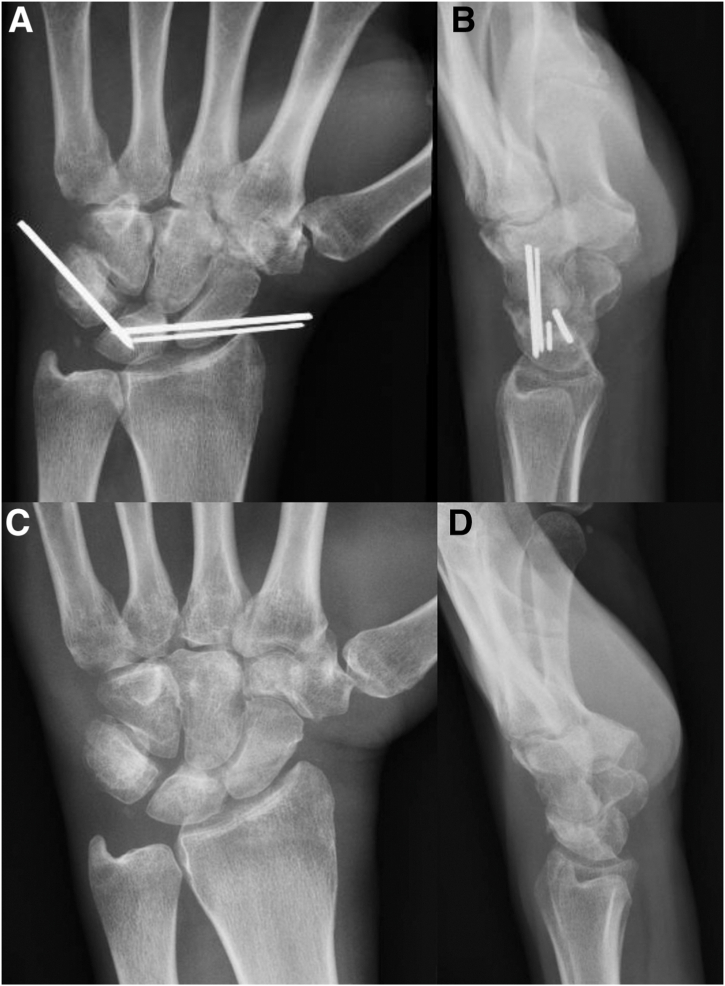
PA and lateral radiographs of our patient with an acute lesser arc perilunate injury 2 weeks postoperatively in fiberglass cast (A and B), with subsequent removal of buried K-wires in clinic 8 weeks postoperatively. (C and D) PA and lateral x-rays of patient demonstrating restoration of Gilula’s arcs and maintained SL and LT intervals, 3 months postoperatively.
